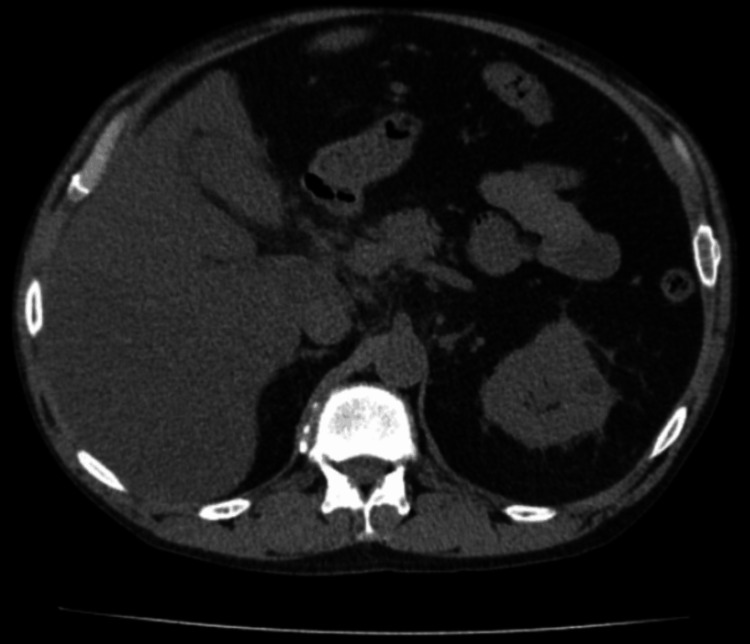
Non-contrast CT of the abdomen and pelvis revealing hepatic steatosis and misty mesentery in the RUQ. RUQ: right upper quadrant
